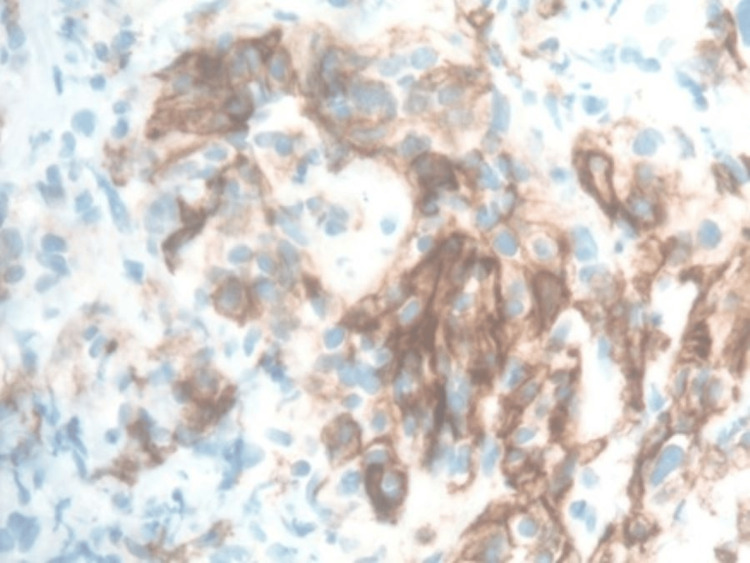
Neoplastic cells are negative for CK7, NKX3.1, PSMA, and RCC. PSMA: prostate-specific membrane antigen; RCC: renal cell carcinoma.
